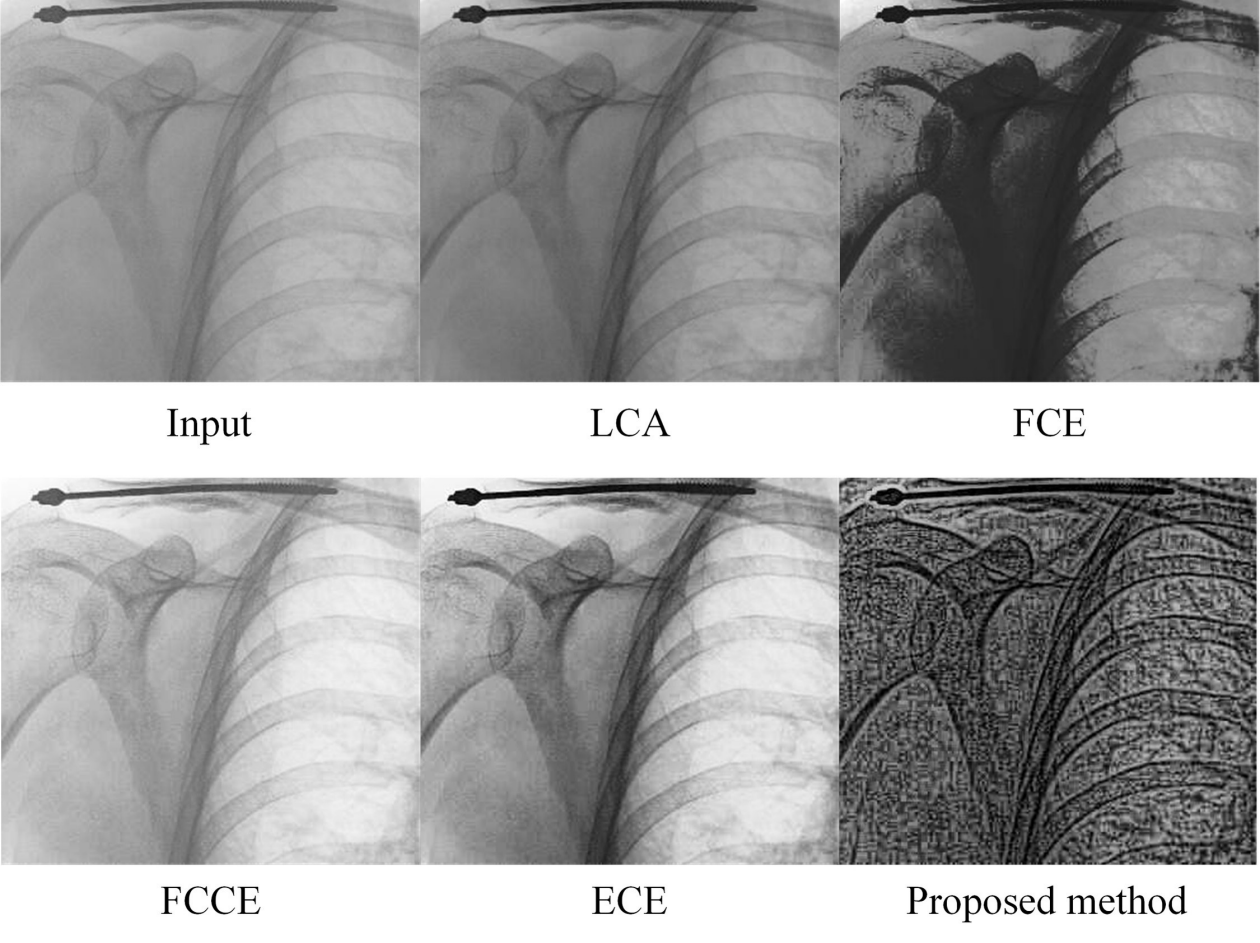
Our proposed enhancement technique exhibits enhanced performance in elevating X-ray image quality relative to established methodologies, including LCA [47], FCE [44], FCCE [43], and ECE [41].
